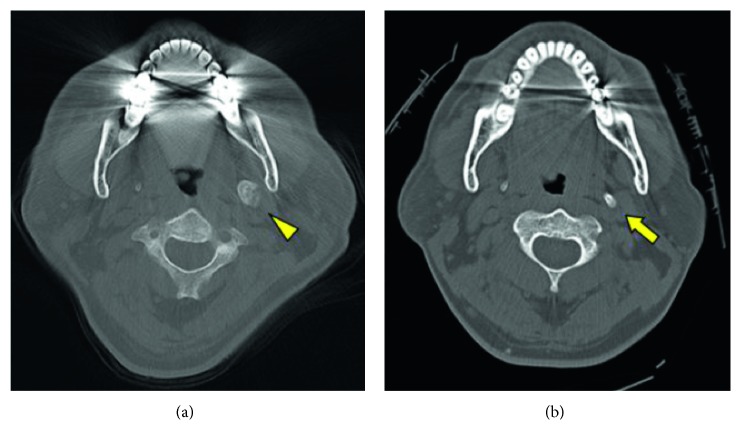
(a) Preoperative computed tomographic image showing enlargement of the left styloid process to approximately 10 mm thick (arrowhead). (a) A computed tomographic image taken 5 years earlier showing enlargement of the styloid process to around 5–7 mm thick (arrow) when the patient was examined for a facial injury.
